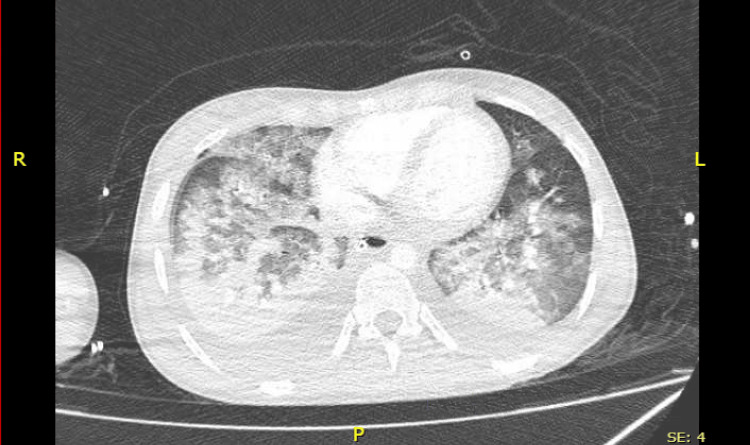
CT angiography showing evidence of pulmonary edema and bilateral moderate pleural effusions.
